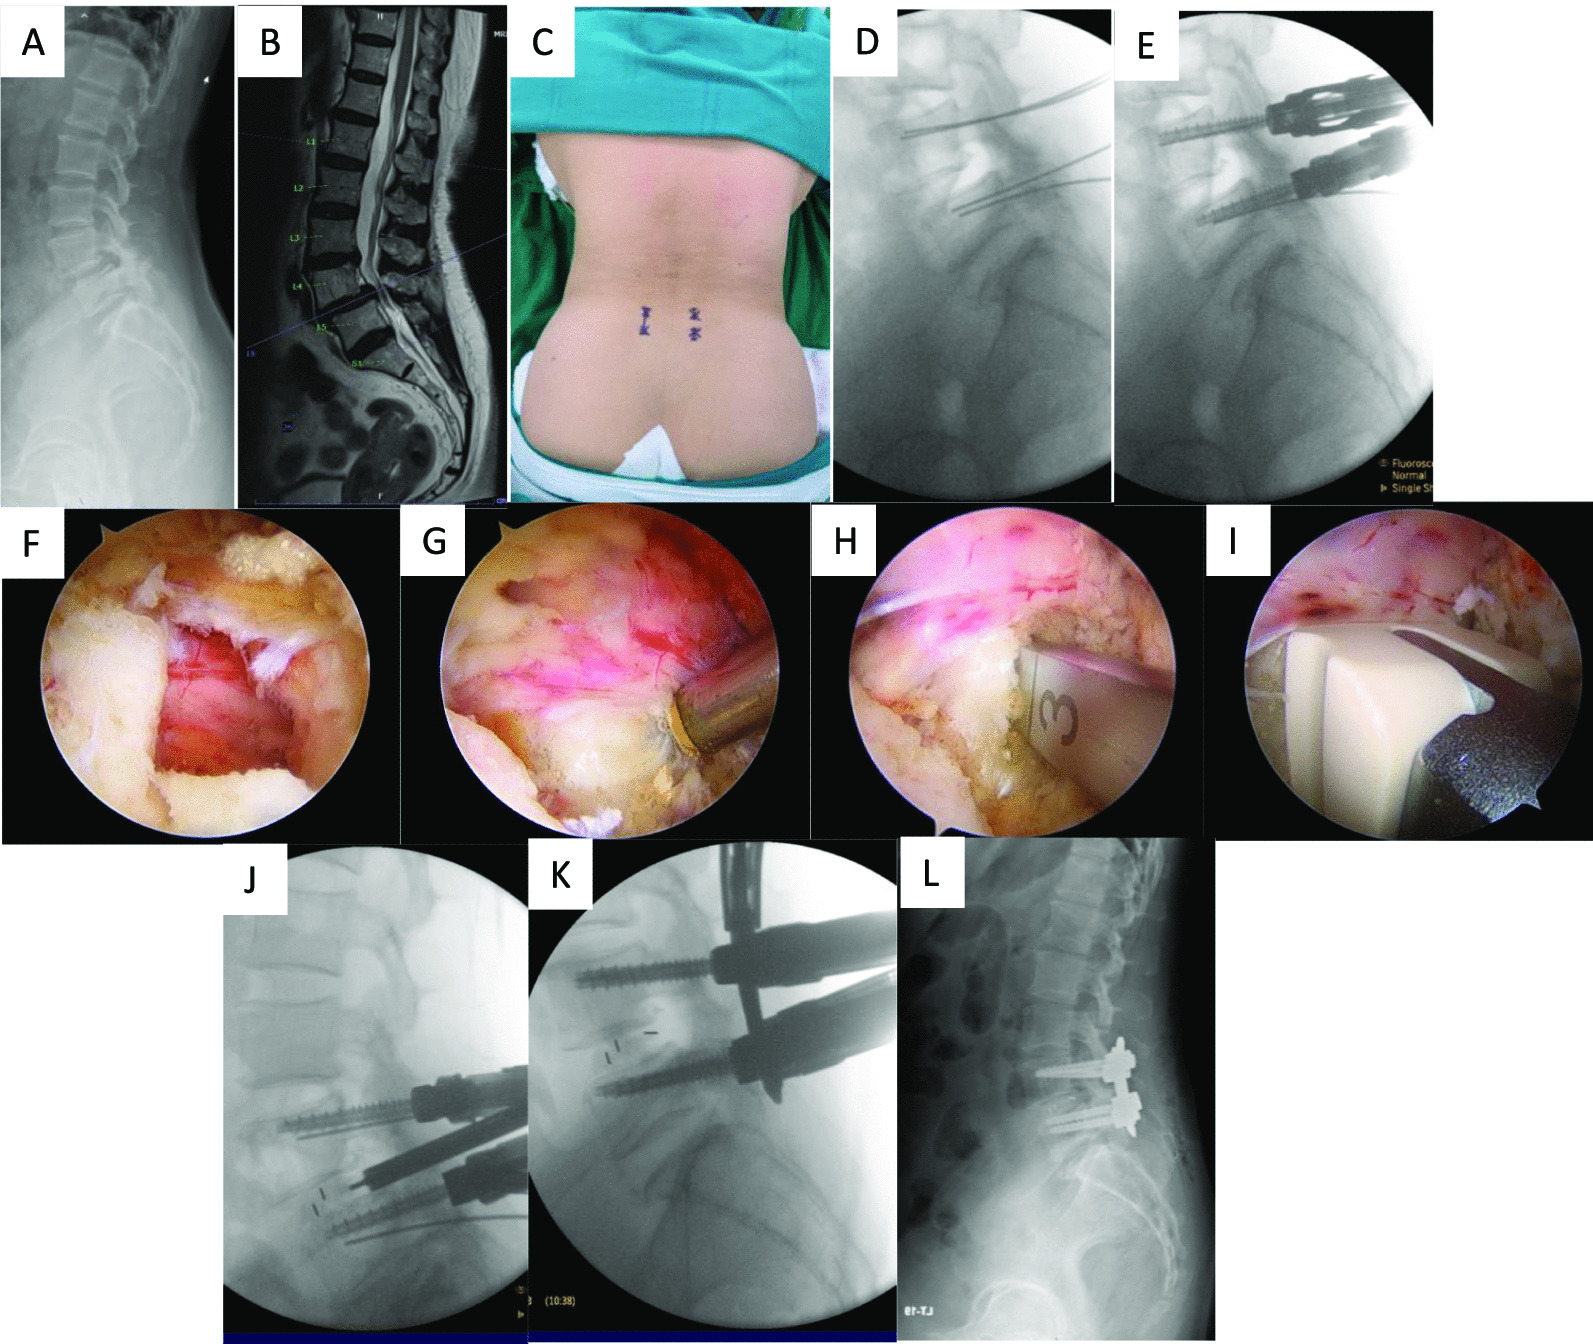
Case demonstration of Endo-TLIF A: Preoperative plain radiograph revealed low-grade spondylolisthesis of L4/L5. B: MRI revealed spinal stenosis at L4/L5. C: Skin incision marked. D: Intraoperative fluoroscopy revealed the guide wire was inserted into the upper and lower pedicle. E: Contralateral pedicle screw was inserted. F: Intraoperative endoscope revealed the laminectomy was done, and the ligamentum flavum was removed. G: The intervertebral disc was identified. H: Intervertebral disc was removed by disc shaver. I: The intervertebral cage was inserted into the intervertebral disc space. J: The position of the intervertebral cage was con-firmed with fluoroscopy. K: The pedicle screws were connected by a rod. L: Postoperative plain radiograph after L4/L5 Endo-TLIF
